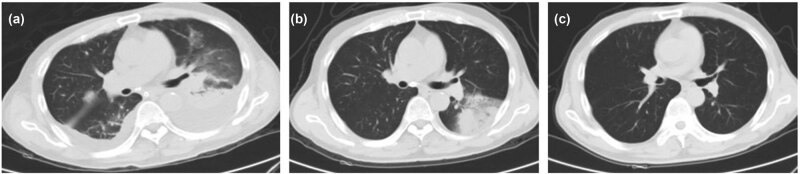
The CT image of case 2. Picture (a) (2020.12.18) is the admission D1, pleural effusion in both lungs, exudation, consolidation, and exudation in the lower lobe of the left lung. (b) Picture (2020.12.29) is the admission D12, Dorsey cyclin D4, the pleural effusion in both lungs was completely absorbed, and the lesions in the lower lobe of the left lung were significantly reduced. Picture (c) (2021.03.11) was reviewed 2 months after discharge, and no obvious lesions were found in both lungs.
